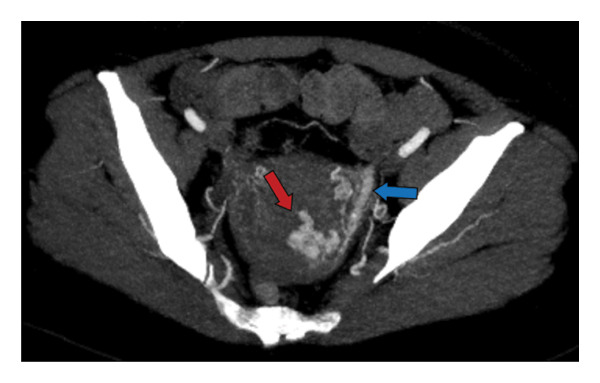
Maximum intensity projection (MIP) axial‐view computed tomography (CT) angiogram of the pelvis demonstrating multiple enhancing left uterine artery branches (red arrow) with early venous drainage (blue arrow), consistent with AVM.
